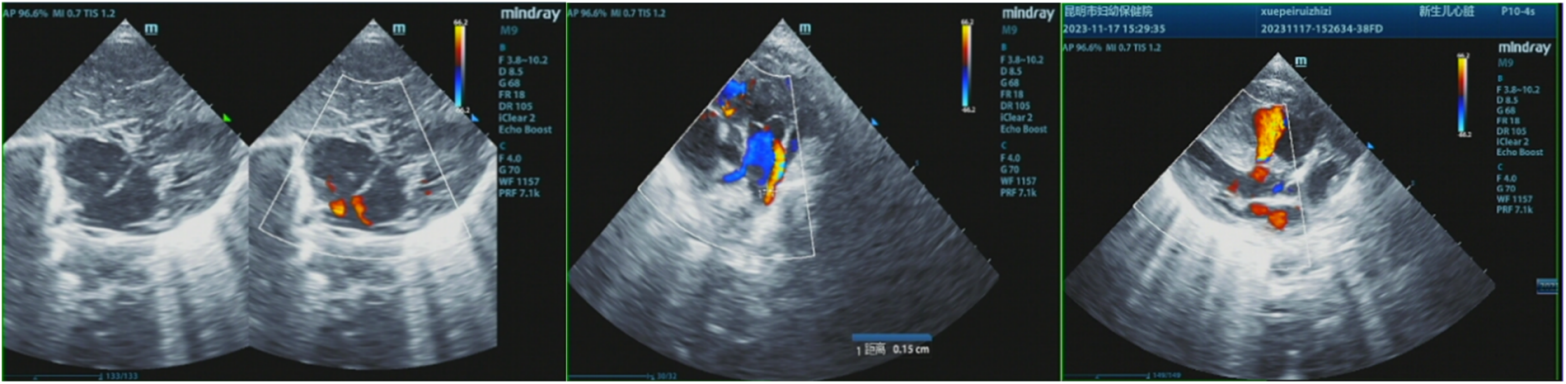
The postnatal echocardiogram results of the patient showed a VSD (6 mm, membranous) with predominant left-to-right bidirectional shunting at the ventricular level, an ASD (5 mm, central secundum) with left-to-right shunting at the atrial level, a PDA (1.5 mm) with predominant left-to-right bidirectional shunting at the arterial level, relatively narrow diameters and increased flow velocity in the bilateral pulmonary arteries, significant thickening of the interventricular septum and right ventricular anterior wall, and moderate to severe pulmonary hypertension (PH).
